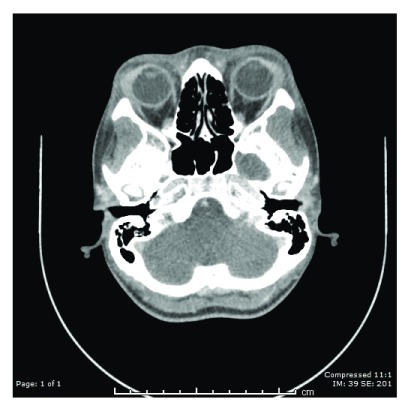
Computed tomography (CT) scan coronal view head with contrast. Bilateral lacrimal glands appear large with mild increased enhancement.
